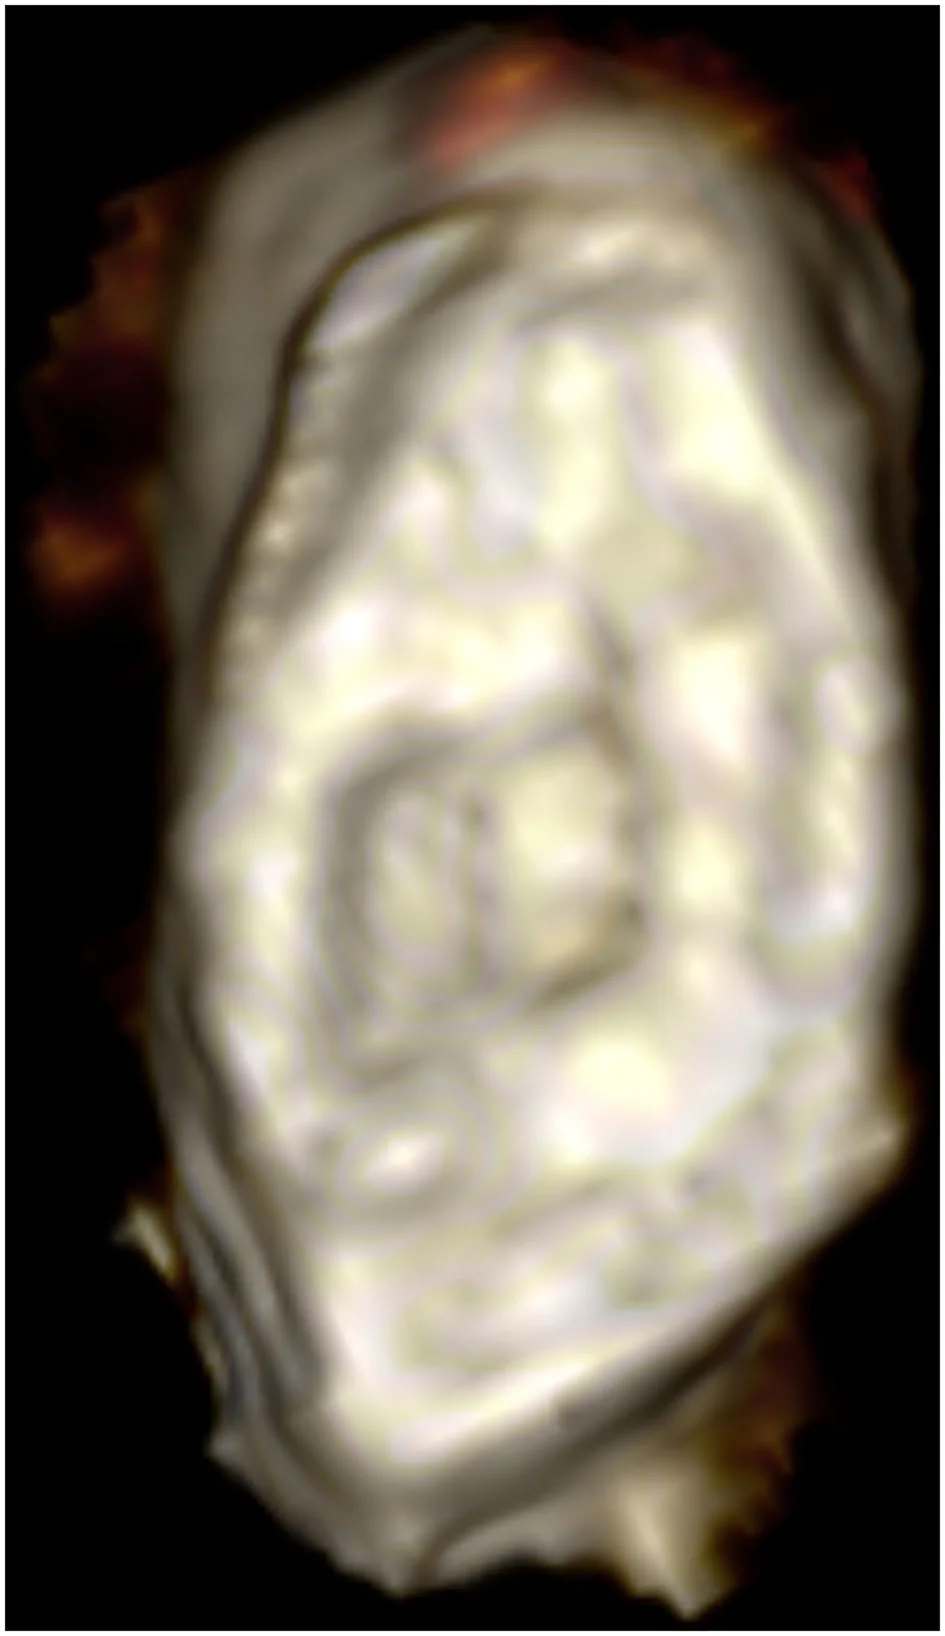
Three-dimensional CT image of the surface of the symphysis pubis of Amenhotep I the morphology indicates stage 4 corresponding to 35.2 ± 9.4 years old.
